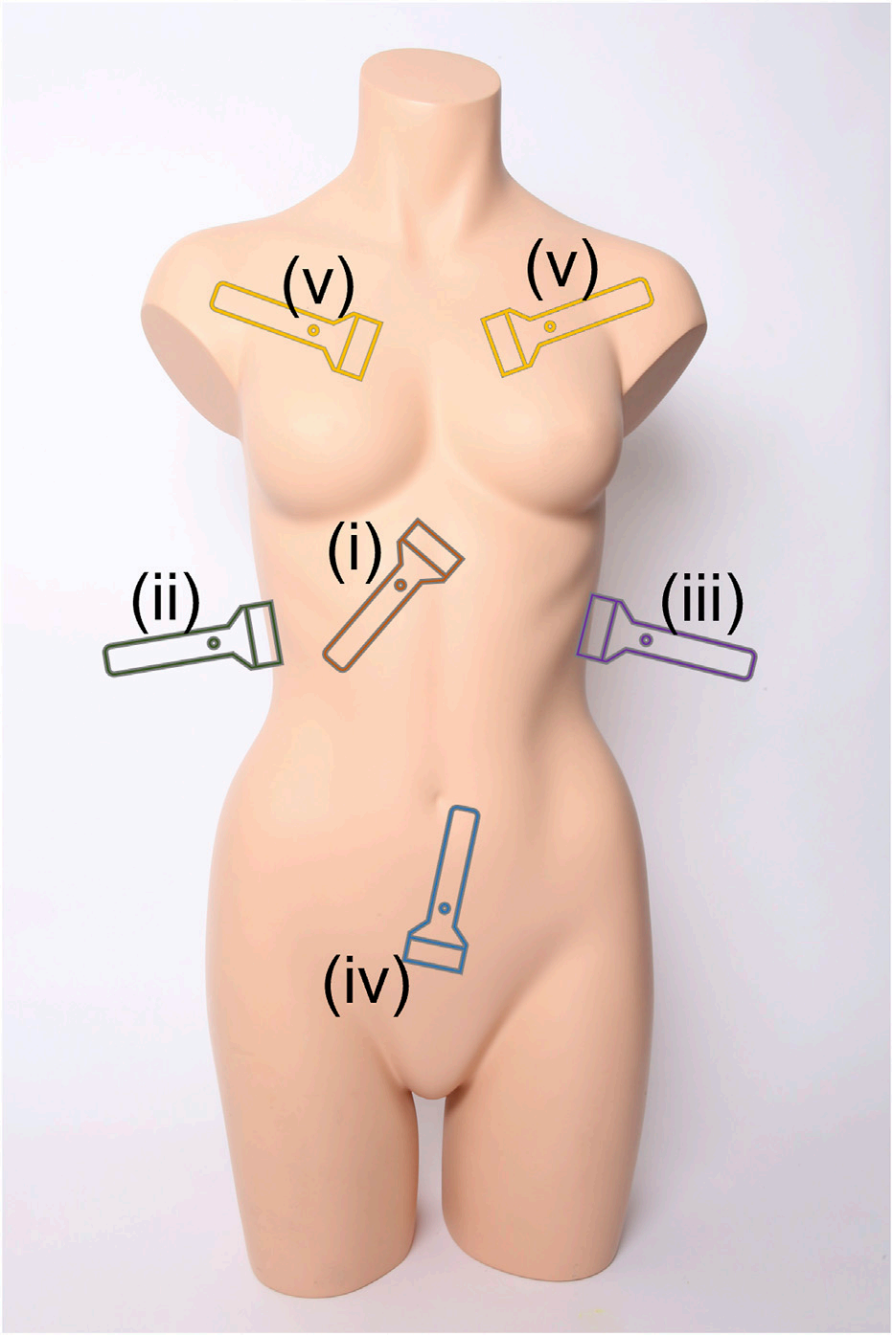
Ultrasound scan points for the extended Focused Assessment with Sonography for Trauma (eFAST) exam. Views include (i) subxiphoid, (ii) right upper quadrant, (iii) left upper quadrant, (iv) pelvic, and (v) intercostal scan points. Image obtained from creative commons as: “Torso” by Tim Reckmann | a59.de is licensed under CC BY 2.0 DEED and was modified to include eFAST scan location markers.
